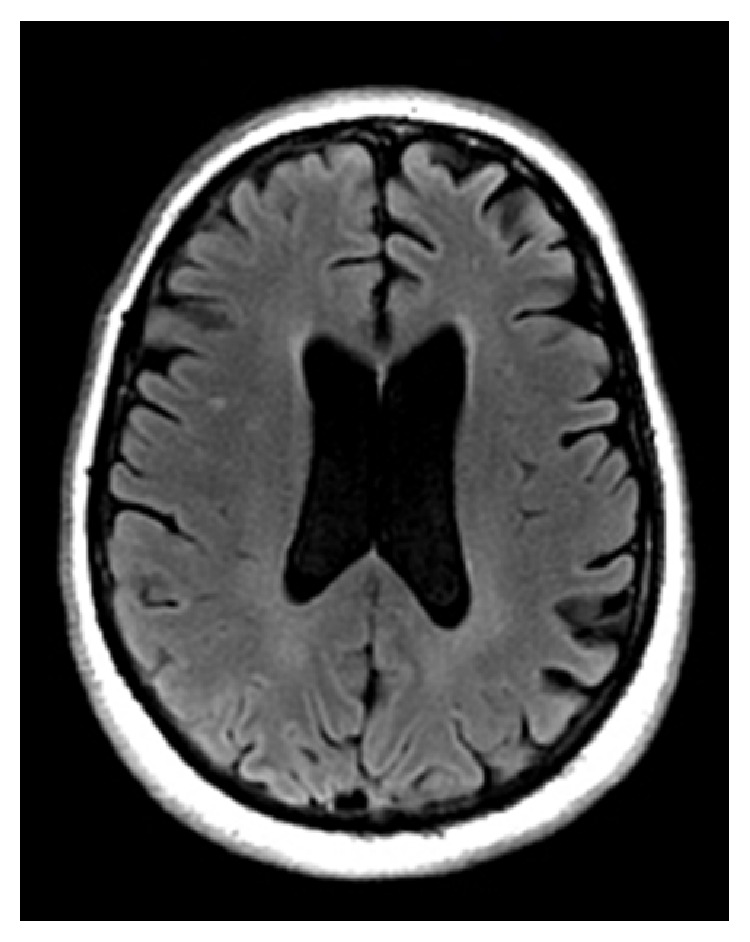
Patient's MRI revealing left thalamic infarct and chronic small vessel ischemic changes.
